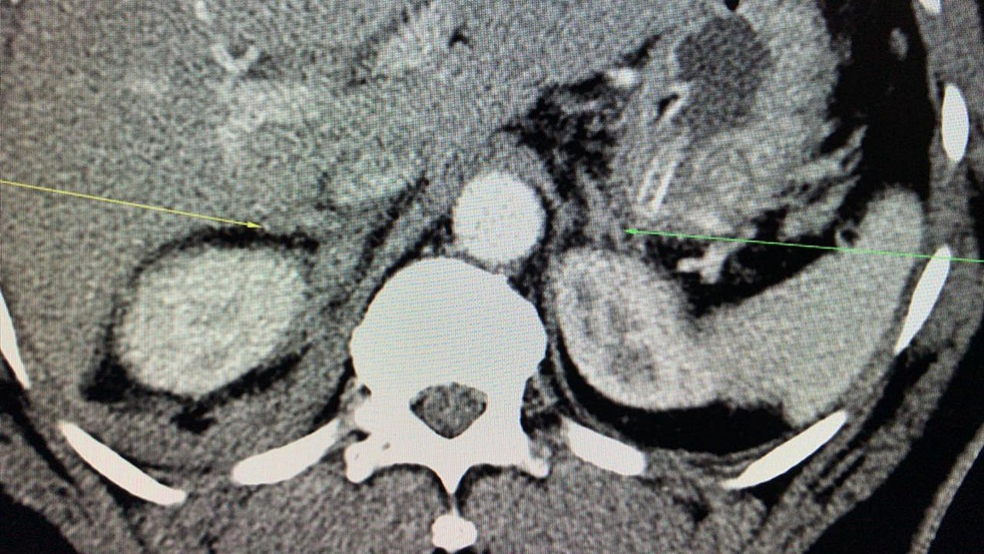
CT scan of abdomen and pelvis with intravenous contrast showing contused right adrenal gland ( yellow arrow) and normal left adrenal with fatty hilum (green arrow)
